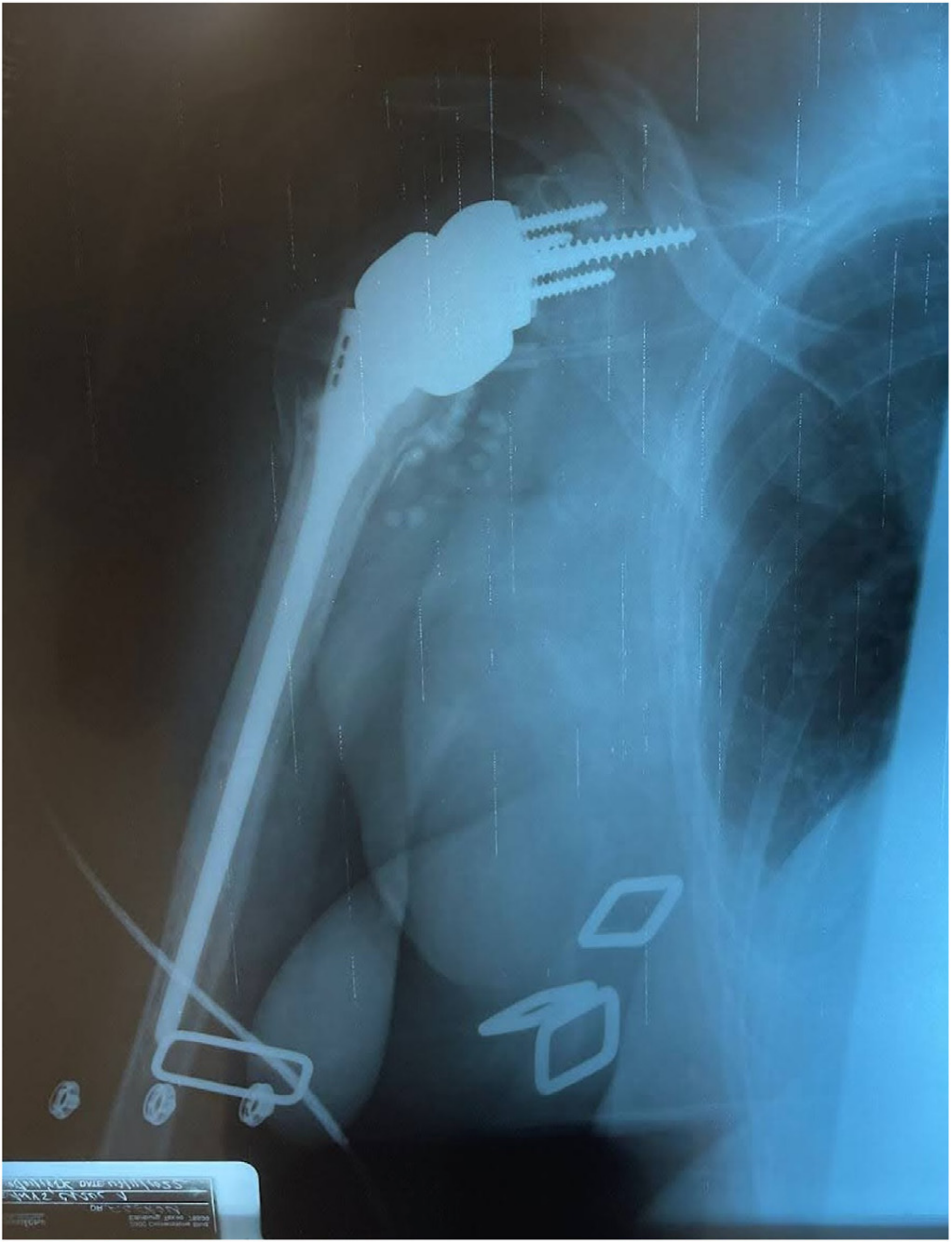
Immediate postoperative radiographs following distal clavicle resection for large central glenoid defect and adequate glenoid baseplate fixation.
